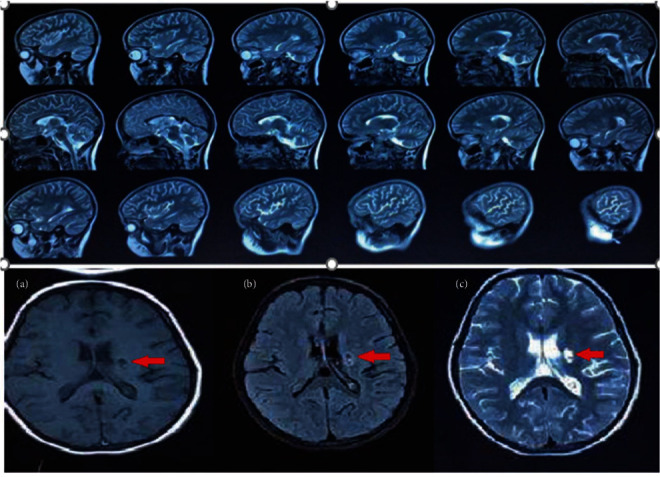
Sagittal T-2 weighted MRI images show mild cerebellar atrophy and brainstem involvement. Axial T-1 weighted (a), gad enhanced (b), and T-2 weighted (c) images of brain MRI demonstrate a focus of left internal capsule infarction (red arrows).
